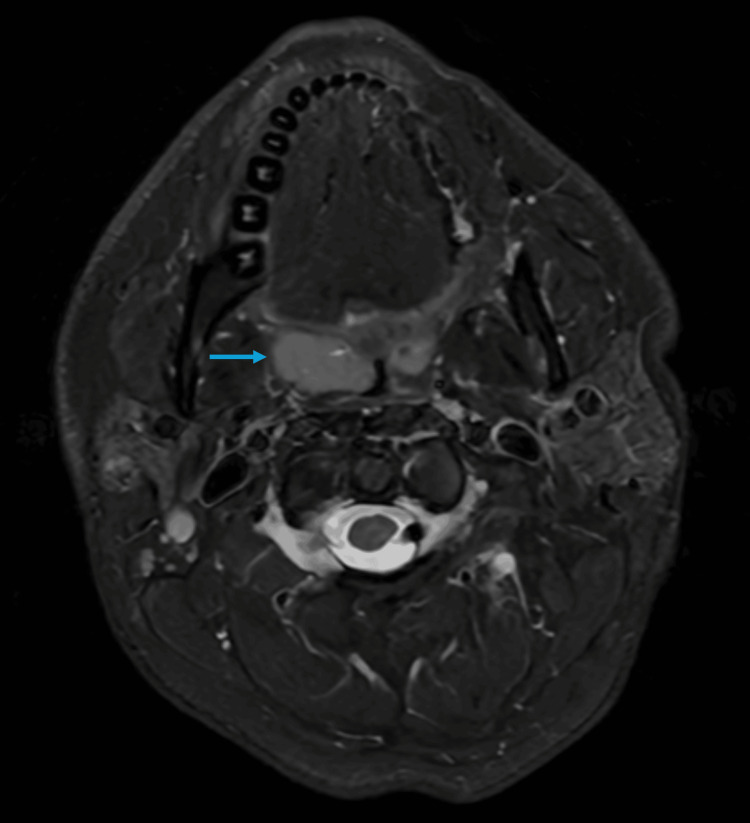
Asymmetric tonsillar appearance on contrast-enhanced MRI Contrast-enhanced axial MR image of the neck demonstrating asymmetric tonsillar enlargement, with the blue arrow indicating the enlarged right tonsil and no evidence of local invasion. MRI: magnetic resonance imaging
